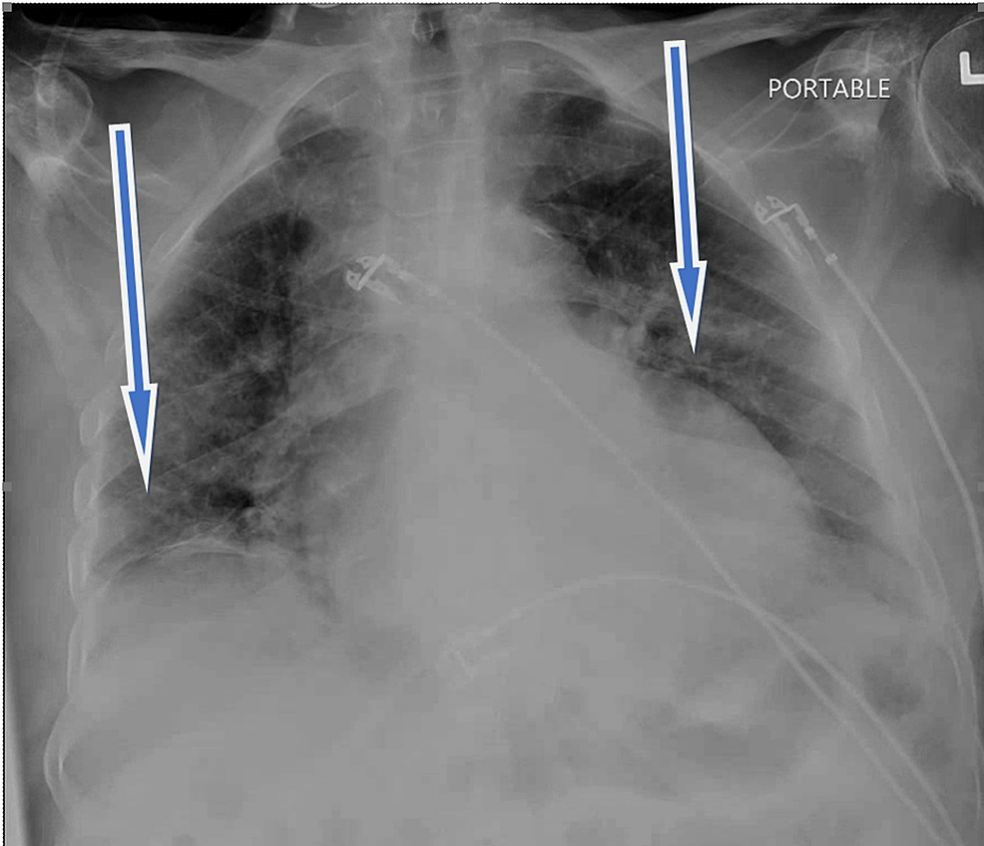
CXR showing bilateral infiltrates (blue arrows) CXR: chest X-ray
